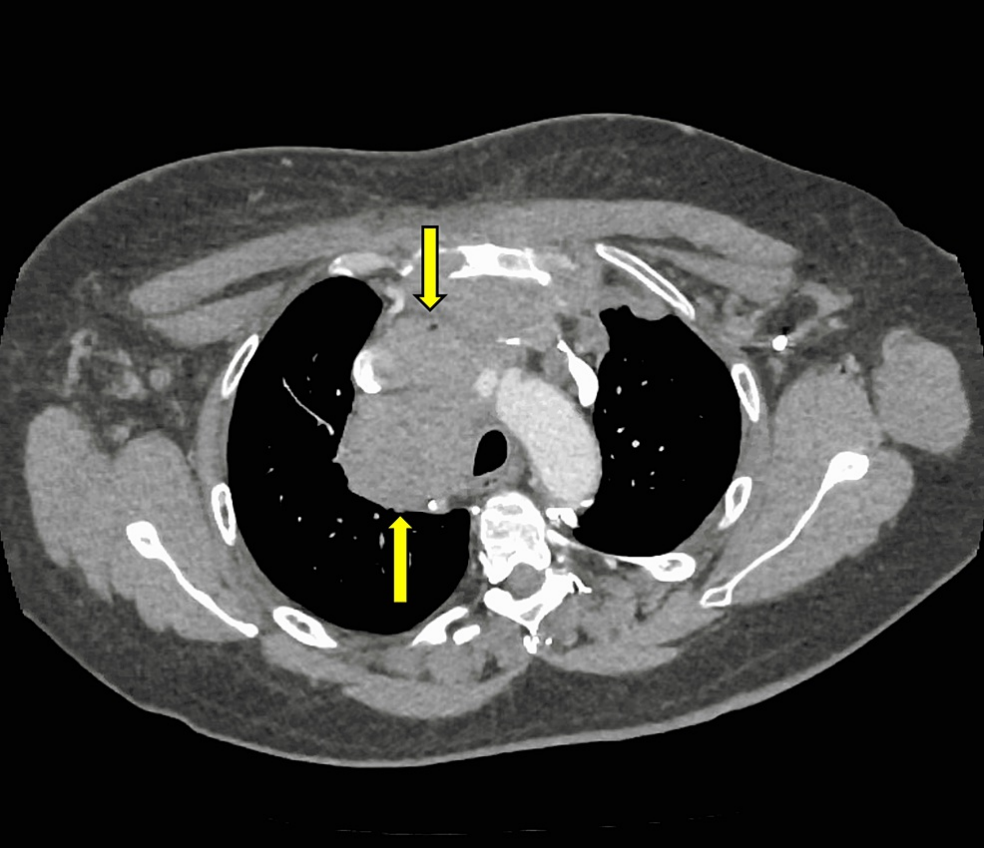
Axial CT thorax image demonstrating an aggressive anterior mediastinal mass. The mass overlaid the sternum and manubrium and was locally invasive, obstructing the superior vena cava and the left brachiocephalic vein. The mass also extended into the left pleural space with likely associated invasion to the left chest wall and left upper lobe.
